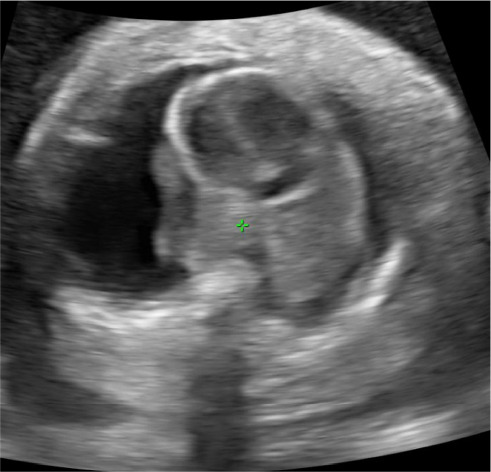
Ultrasound demonstrating bilateral pleural effusions.
